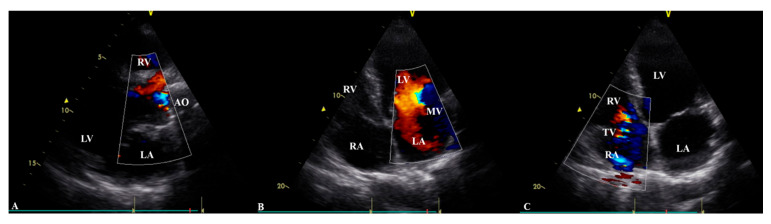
(A) Long-axis parasternal view showing mild (Grade I) aortic valve regurgitation. (B) Apical four-chamber view showing moderate-to-severe (Grade II–III) mitral valve regurgitation. (C) Apical four-chamber view showing moderate (Grade II) tricuspid valve. regurgitation. LV—left ventricle, LA—left atrium, RA—right atrium, RV—right ventricle, AO—aorta, MV—mitral valve, TV—tricuspid valve.
